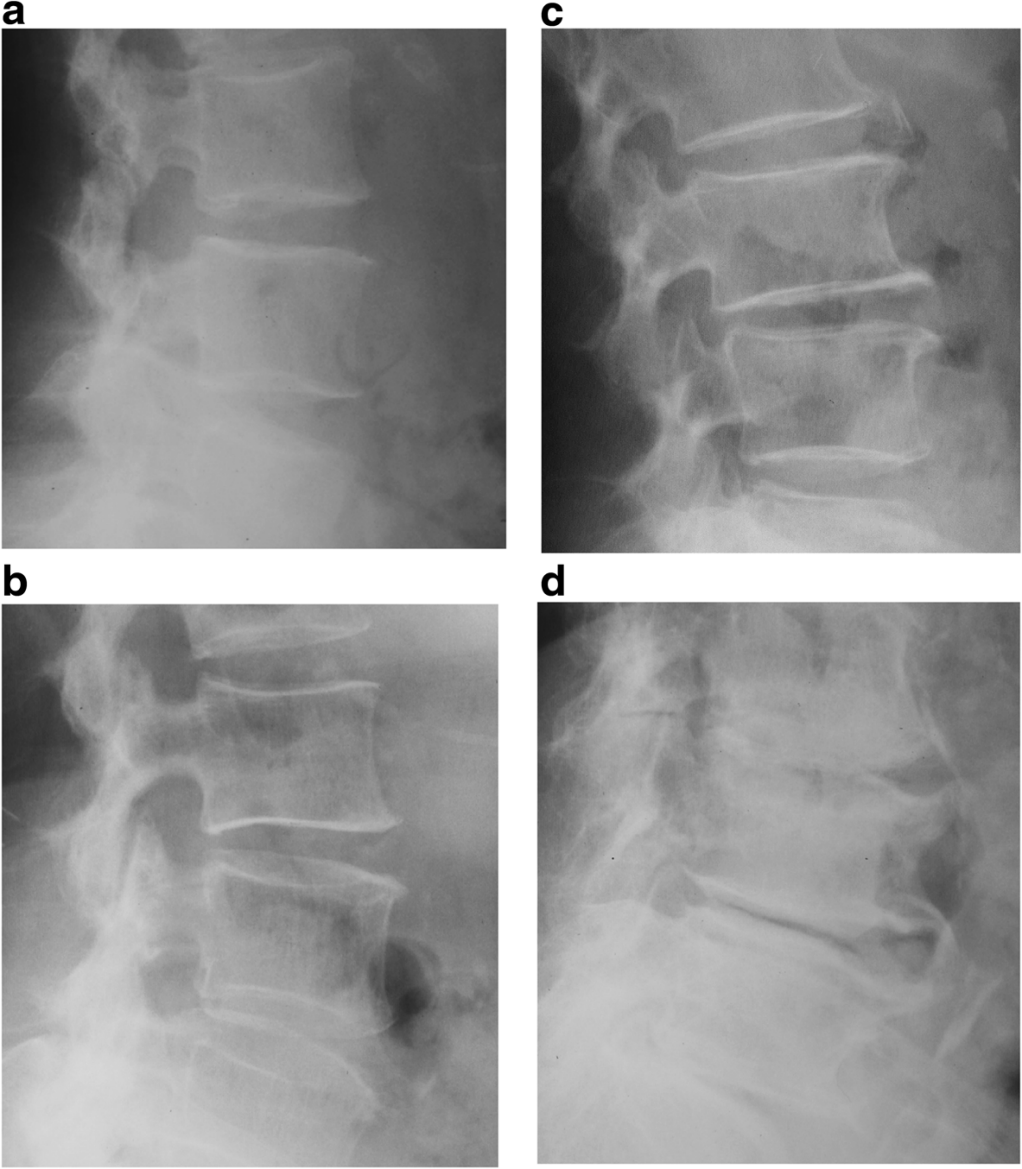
Radiographs were scored for lumbar spondylosis using the Kellgren-Lawrence (KL) grade as follows: KL1, slight osteophytes (a); KL2, definite osteophytes (b); KL3, disc space narrowing with osteophytes (c); KL4, bone sclerosis, disc space narrowing, and large osteophytes (d)
